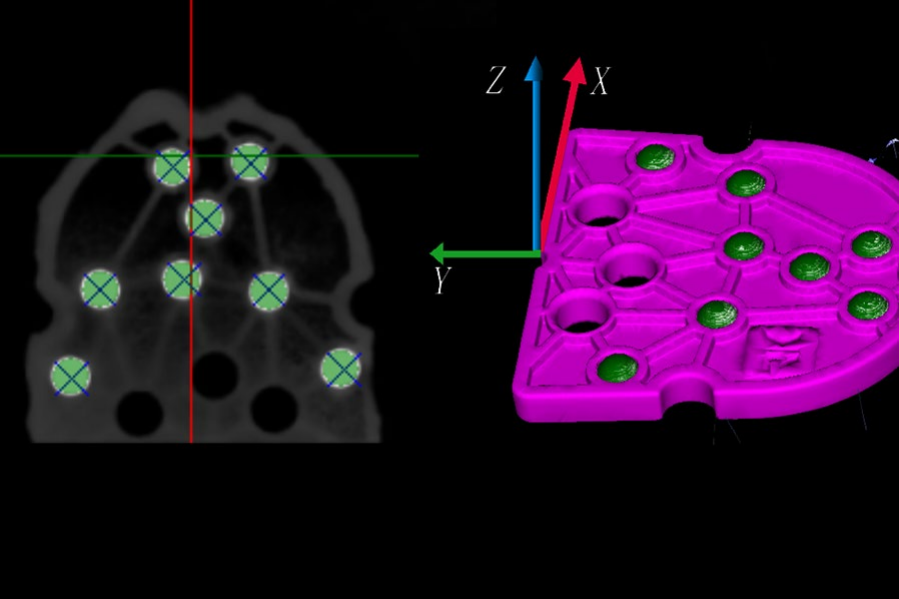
Coordinate alignment in the implant planning software. Left: The software identified the zirconia beads in the diagnostic plate in the image. Right: The positions of the diagnostic plate and the radiographic template were aligned with the coordinates of the software
